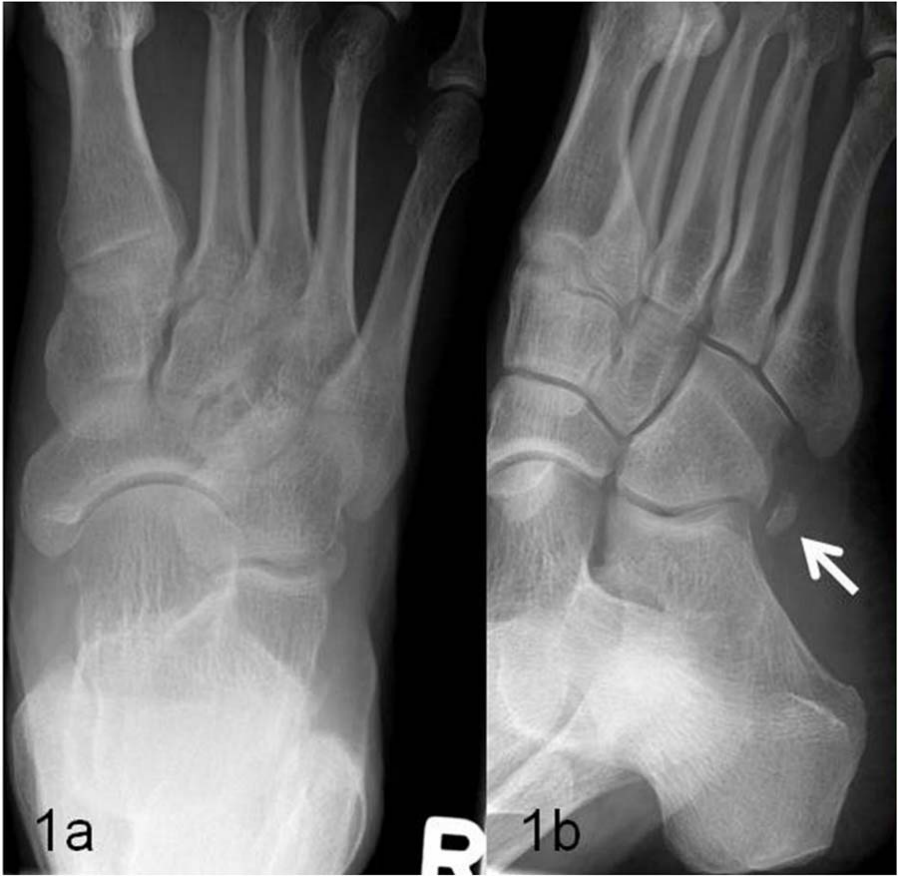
(a, b) Presentation of the os peroneum (marked with an arrow) in the oblique X-ray image.
